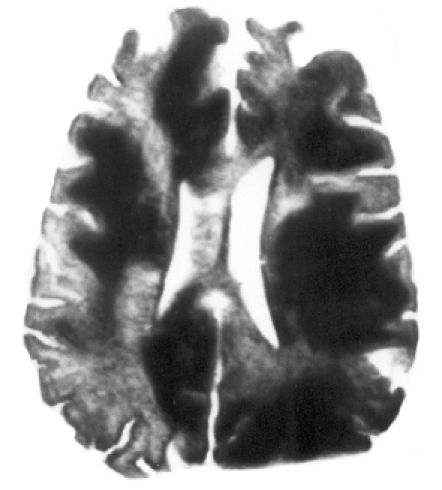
FLAIR axial image at the level of the body of the lateral ventricle. Confluent hyperintense lesions are seen in the subcortical white matter with involvement of ‘u’ fibres bilaterally in an asymmetrical distribution.
